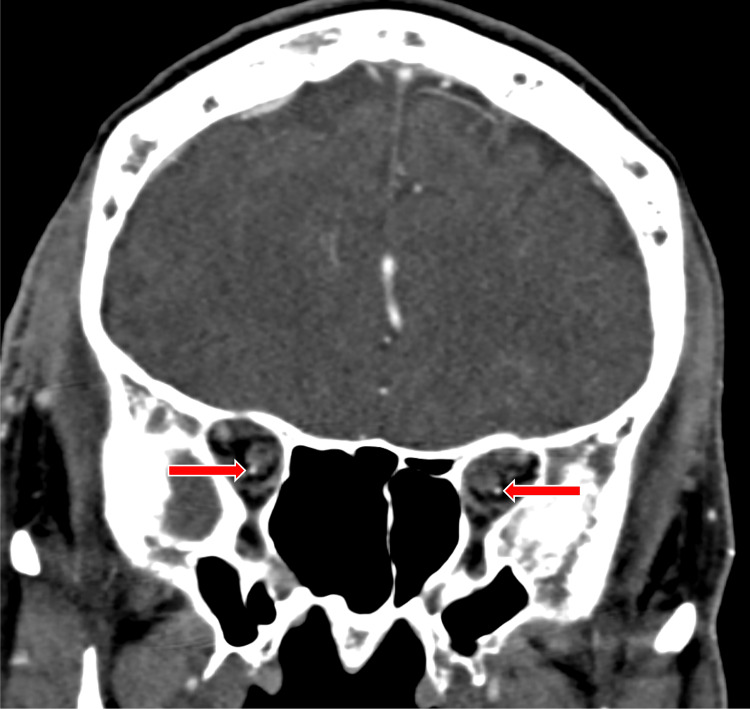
Coronal CTA image The computed tomography angiography (CTA) image demonstrates patent ophthalmic arteries bilaterally in the orbits (red arrows).
